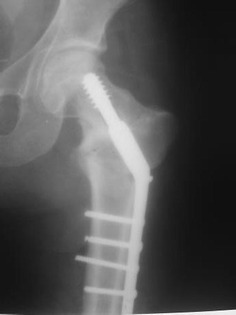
Radiograph showing the final picture in a patient operated for coxa vara
